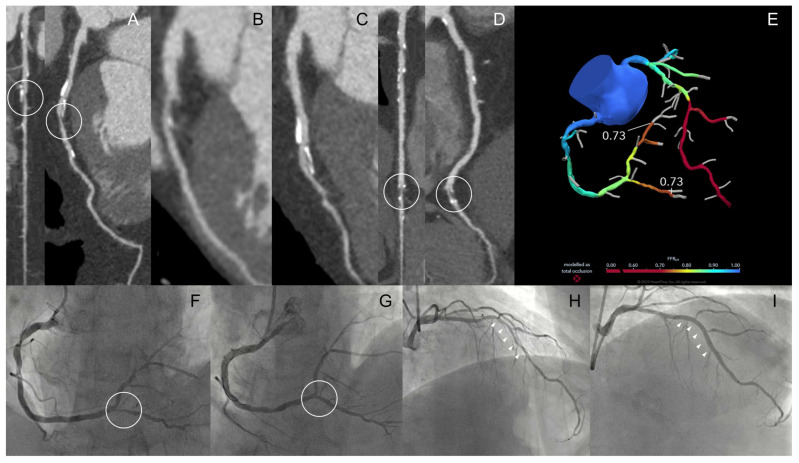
A 63-year-old man, smoker, known for diabetes, hypertension and dyslipidemia presented with effort chest pain (walking), resolved at rest. CCT showed severe stenosis of the left anterior descending artery (white circles; (A)), mild disease of the first (B) and second (C) diagonal branches and severe stenosis of the right coronary artery-posterior descending artery (white circles; (D)). FFR-CT confirmed the functional significance of both severe stenoses (E). The patient underwent percutaneous coronary intervention and drug-eluting stent implantation on the right coronary artery-posterior descending artery (white circles, (F,G)) and left anterior descending artery (white arrowheads; (H,I)).
